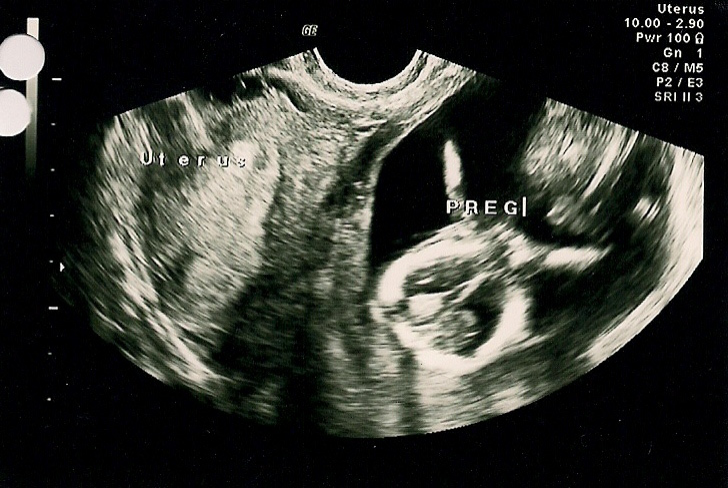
Ultrasonography picture at 14 weeks gestation showing a single fetus, corresponding to date in size, and the possibility of a bicornute uterus.
